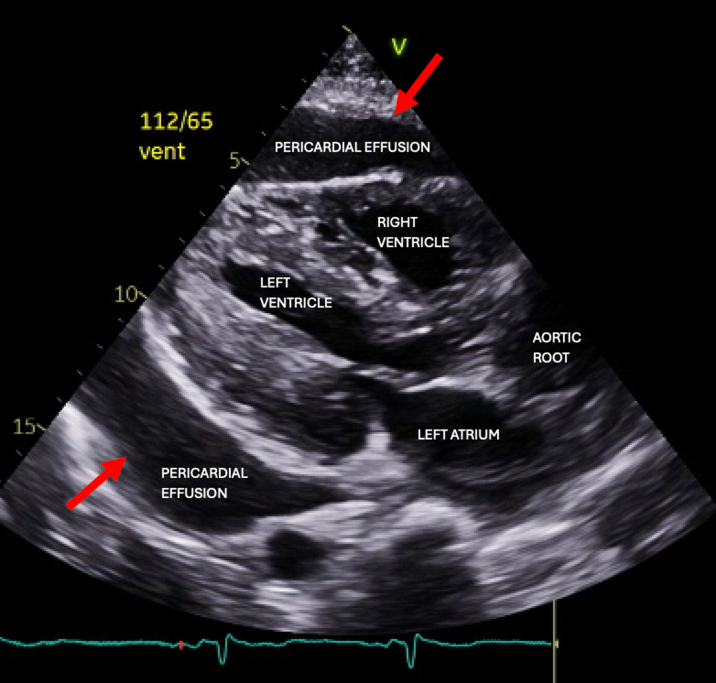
Pretreatment Echocardiogram Transthoracic echocardiogram parasternal long axis view showing moderate pericardial effusion (red arrows) without tamponade.
